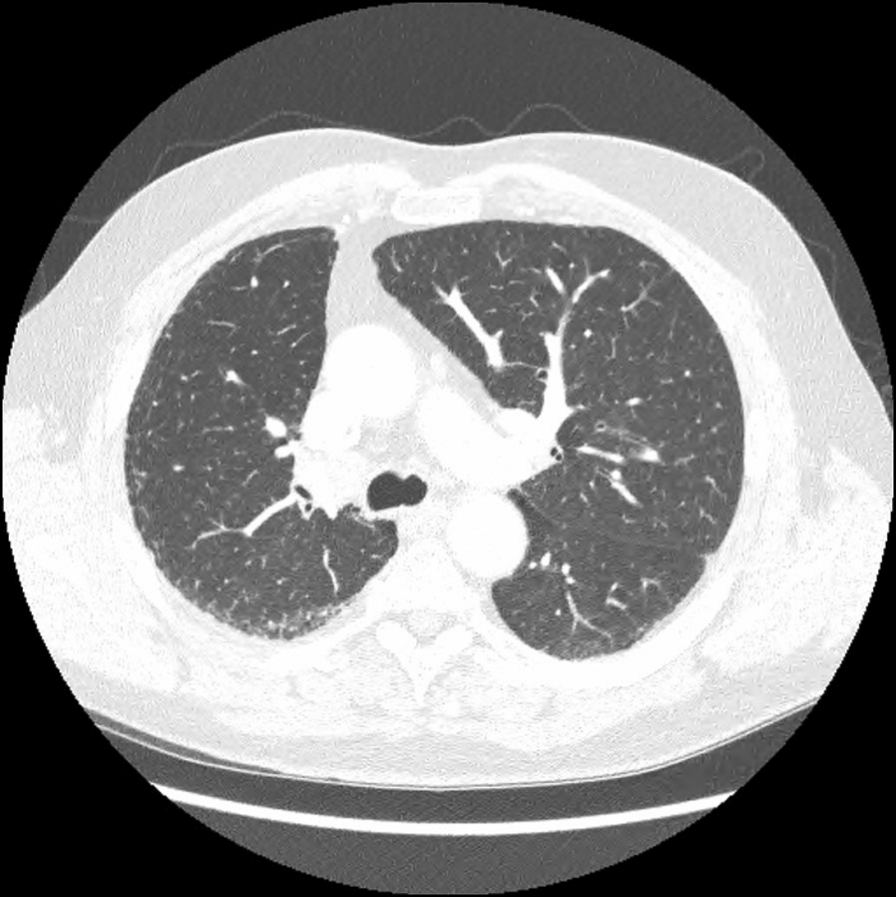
Case 2—CT chest lower lobes revealing signs of pneumonitis, widespread mixed ground glass and interstitial infiltrate. There is progression of malignancy
